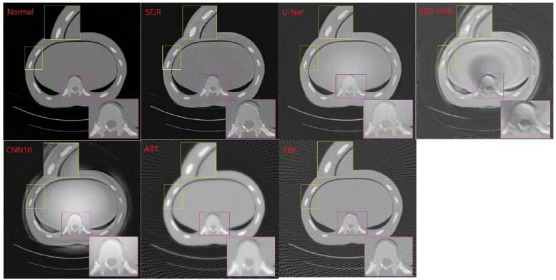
Two regions of interest (ROIs) from the visual differences of the 20% target dose CT image in the FDA dataset reconstructed by SEJR and other methods were selected for detailed comparison.
